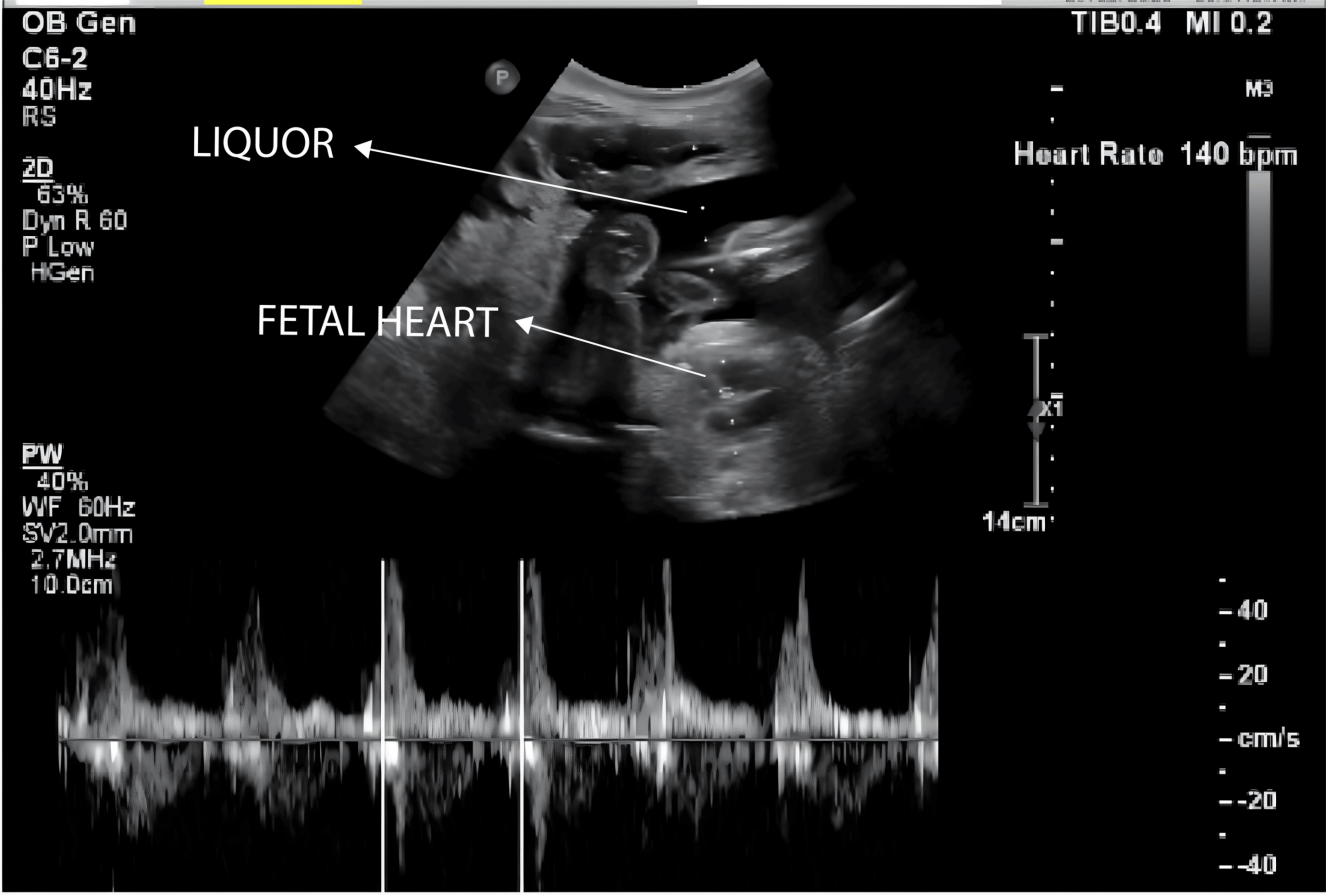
Ultrasound of the abdomen and fetal Doppler of the uterus The image revealed a single live intrauterine gestation (SLIUG) of 26 weeks
